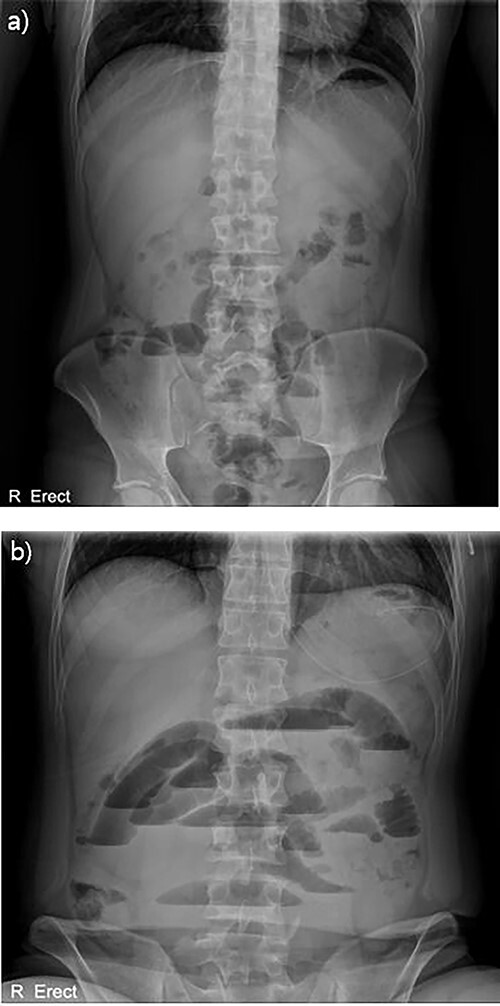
Abdominal X-ray findings (a) initial findings before admission; (b) 12-h follow-up findings revealing step ladder sign.
